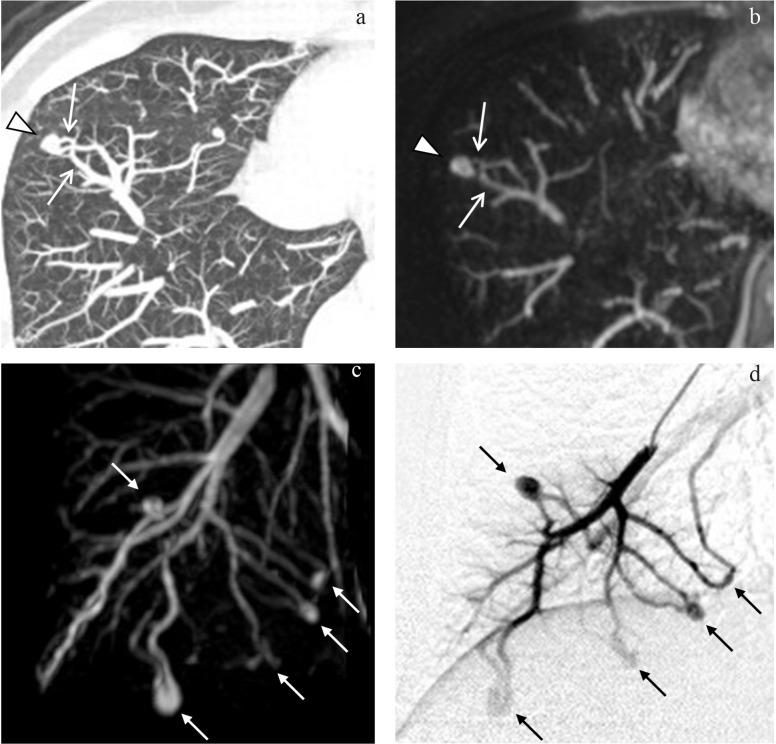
A 22-year-old woman with hereditary hemorrhagic telangiectasia who had multiple pulmonary arteriovenous malformations (PAVMs) in both lungs. (a) Computed tomography (CT) scan (thin-slab maximum intensity projection [MIP]) showed a simple type PAVM in the right lobe (segment 8). Arrows and arrowhead indicate the feeding artery and aneurysmal sac, respectively. (b) On non-contrast-enhanced magnetic resonance angiography with time-spatial labeling inversion pulse (time-SLIP MRA), the feeding artery and aneurysmal sac of the PAVM are clearly visualized, corresponding to those on the CT scan. (c) The MIP image of the time-SLIP MRA in the right anterior oblique view. (d) Multiple simple type PAVMs (arrows) originating from the same segmental artery are seen, which is well in agreement with the findings of digital subtraction angiography (DSA).
